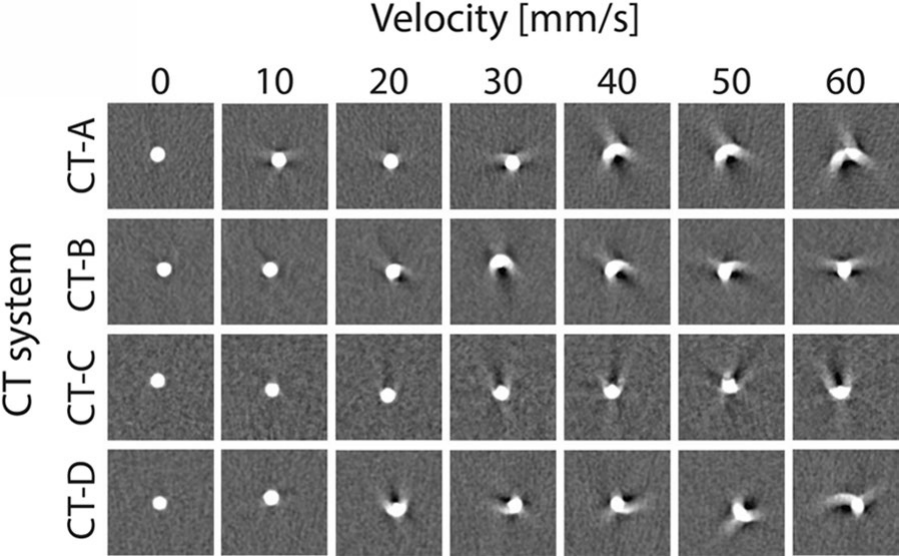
Representative images of the motion artifacts for all CT systems at the minimum and maximum velocity, clinical full and 80% reduced radiation dose and FBP and IR. Window center was 90 HU and window level was 750 HU. *For each CT system, the highest available level of IR was used. *FBP* filtered back projection, *IR* iterative reconstruction
